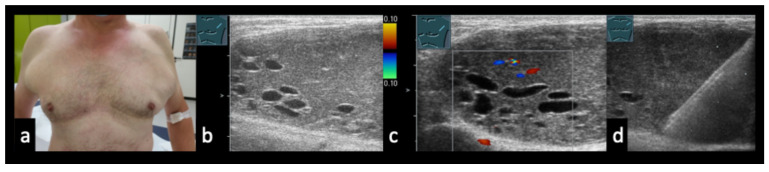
(a) 66-year-old patient with generalized lymphadenopathy. (b,c) B-mode ultrasound (b) and color Doppler sonography (c) show well-demarcated, cystic intranodal lesions without detectable vascularity. (d) Lymph node biopsy revealed lymphocytic lymphoma consistent with B-cell chronic lymphocytic leukemia (B-CLL). No specific comment was made regarding the nature of the cystic areas.
